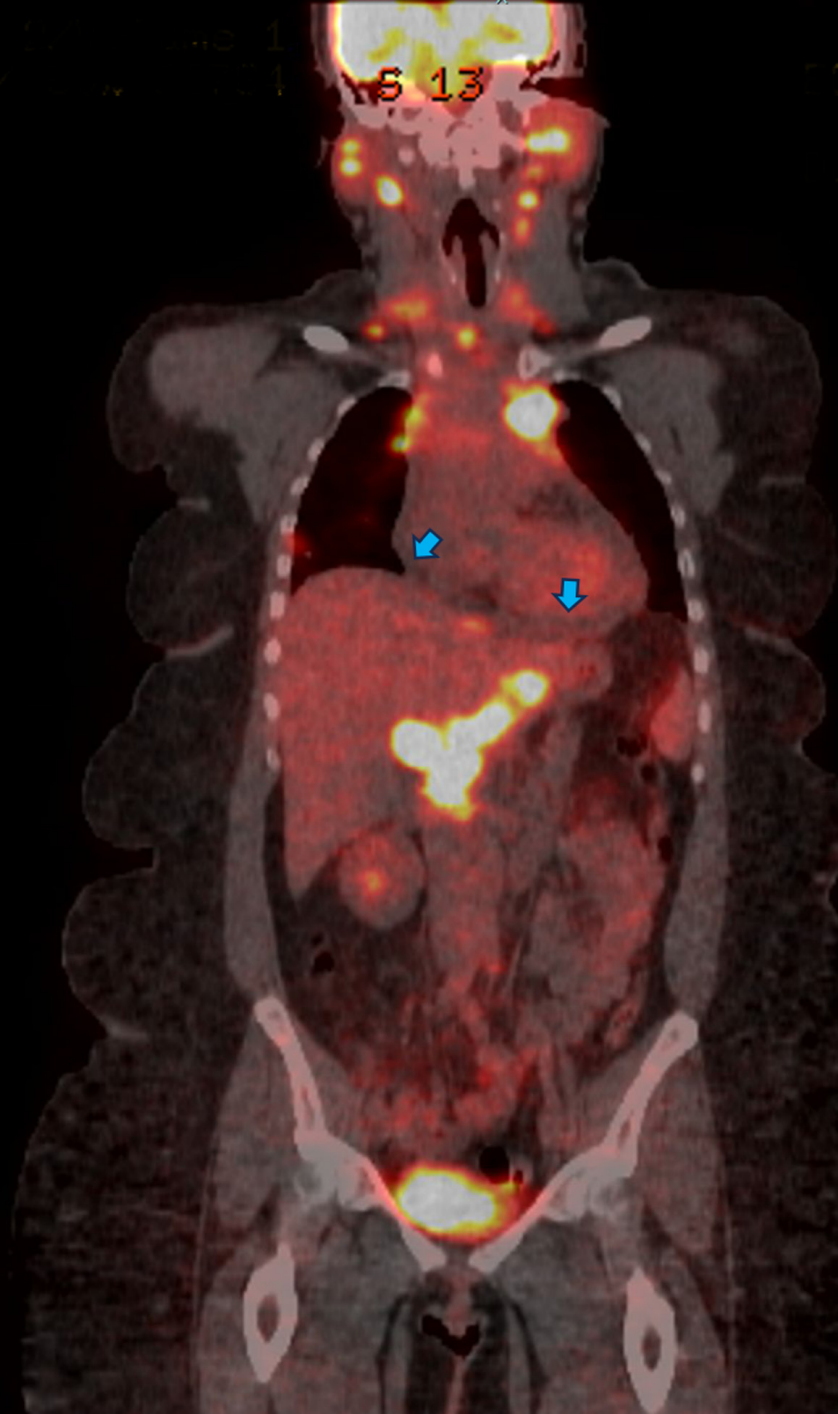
A coronal view of the whole body FDG‐PET fusion scan shows evidence of diffuse lymphadenopathy. The blue arrows indicate a small pericardial effusion with no active metabolization activity.
